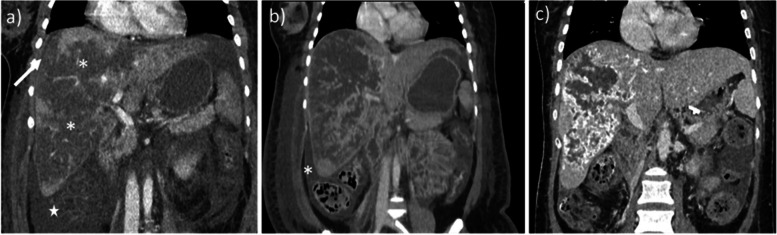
HELLP Syndrome natural history in a 31-year-old woman. Three subsequent scans, all in coronal plane reconstruction, portal venous phase. The first one (a) was held at the end of third trimester, when symptoms first appeared. Liver infarctions (*), ascites (star), and a little subcapsular hematoma (arrow) are seen, typical signs of HELLP syndrome. Immediate delivery was promptly performed after the radiologist’s diagnosis. Control CT scans held after 10 (b) and 40 days (c) show progressive disappearance of subcapsular hematoma and ascites, with calcifications all along the infarcted areas
